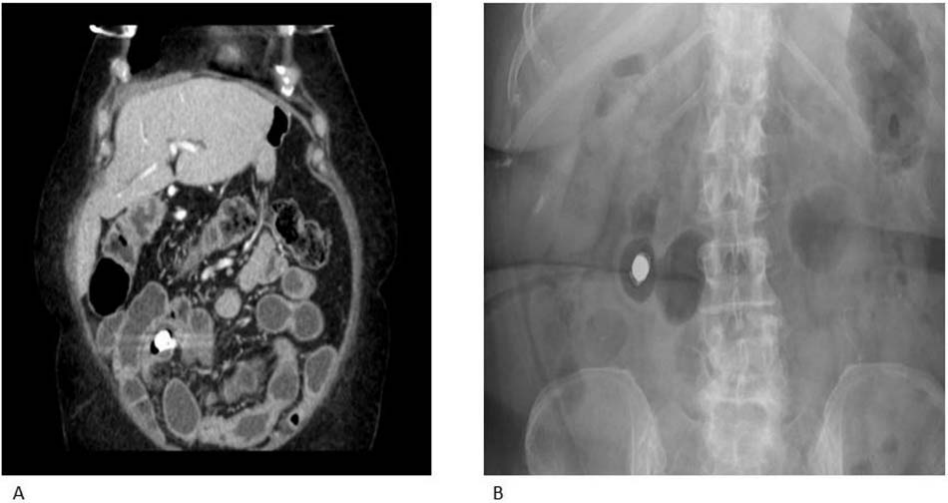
CT enterography (A) and plain X-ray (B) demonstrating multiple areas of mid small bowel stricturing. Some evidence of deep ulceration and skip lesions. Of note, the capsule remains stuck within the small bowel and is at risk of causing obstruction.
